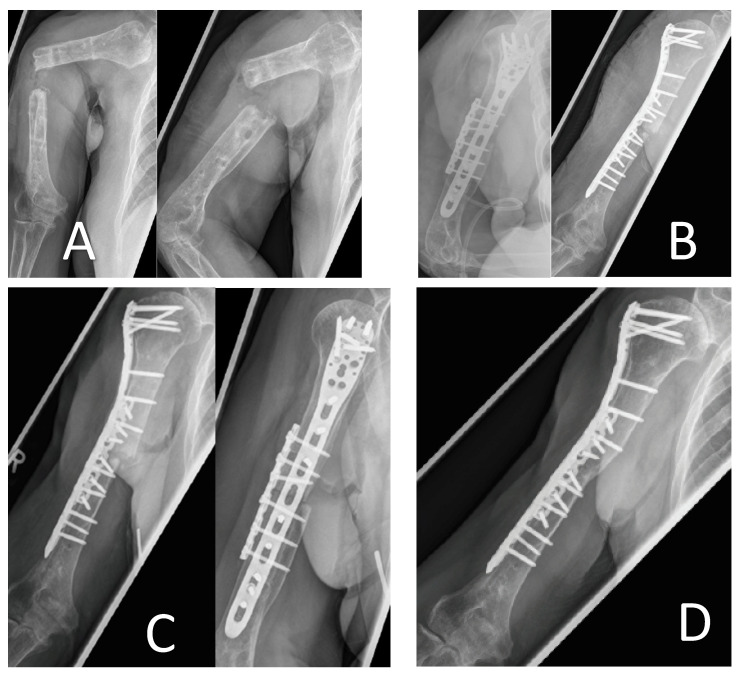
(A) Pre-surgery X-rays; (B) post-surgery X-rays with plate osteosynthesis and defect reconstruction; (C) X-rays at 2 months post-surgery; (D) 1.5 years post-surgery X-rays.
